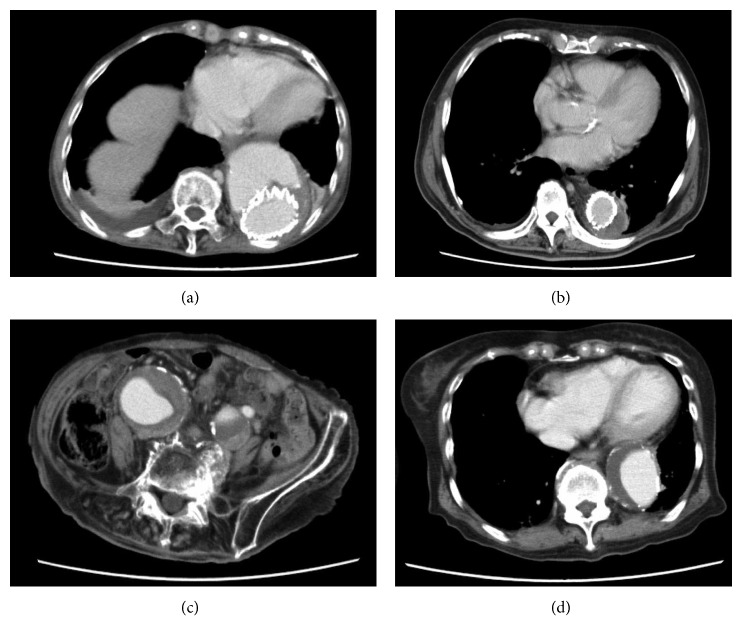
Contrast-enhanced axial CT findings showing aortic aneurysm/dissection in (a) Patient 1, showing a stent graft in the descending aorta; (b) Patient 2, showing a stent graft in the descending aorta; (c) Patient 3, showing aneurysms in the bilateral common and internal iliac arteries; and (d) Patient 4, showing an aneurysm in the descending aorta.
